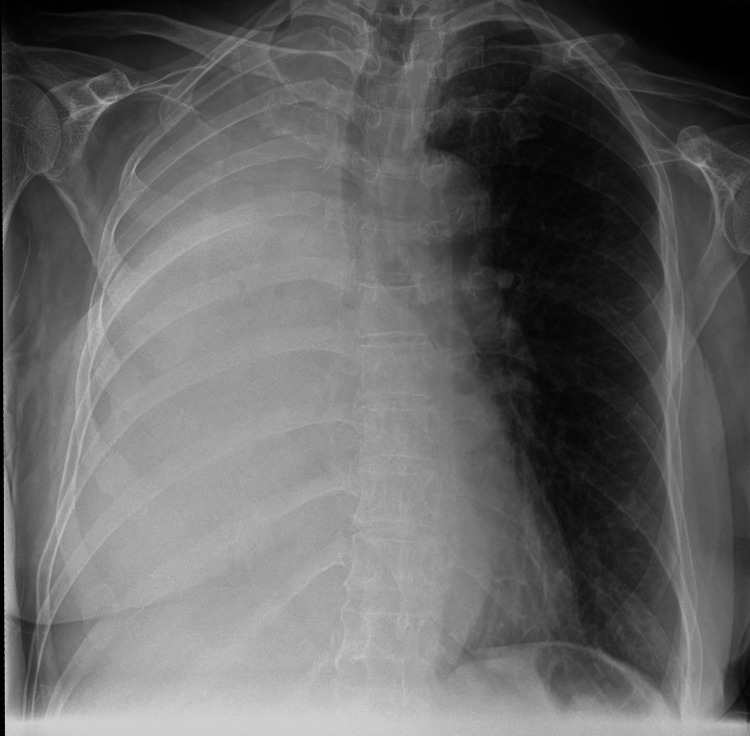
Anteroposterior chest X-ray showing complete whiteout of the right hemithorax due to right total lung collapse
